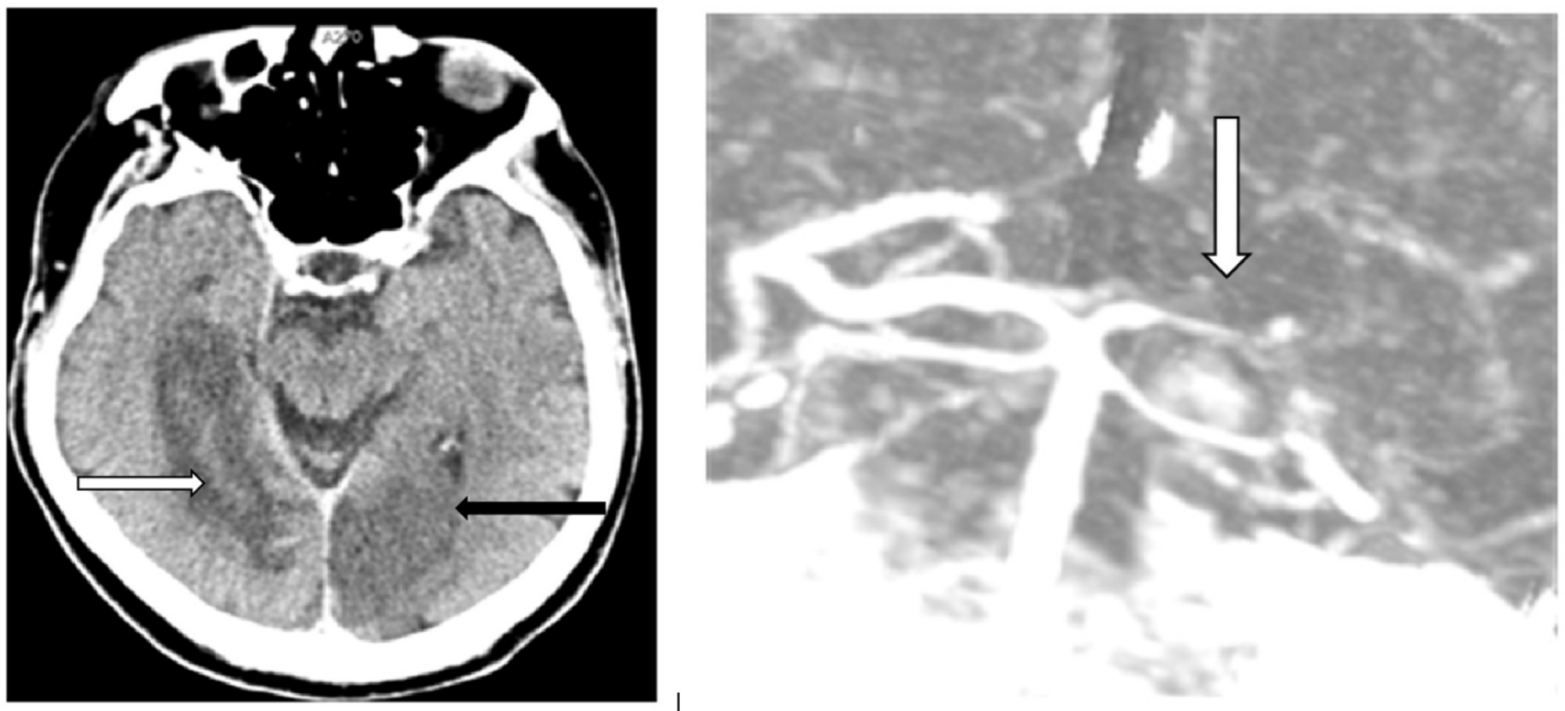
Patient Diagnosed with Anton-Babinski Syndrome Left: Axial computed tomography (CT) brain showing a subacute infarction in the right posterior cerebral artery (white arrow) and a new acute cerebral infarction in the left posterior cerebral artery (black arrow). Right: CT angiography showing the gracile flow of the right posterior cerebral artery (white arrow).
